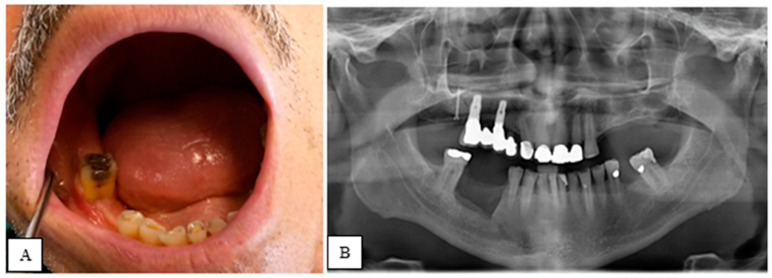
(A) Clinical picture 12 months after surgery (T3); (B) Radiography 12 months after piezoelectric surgery (T3).
